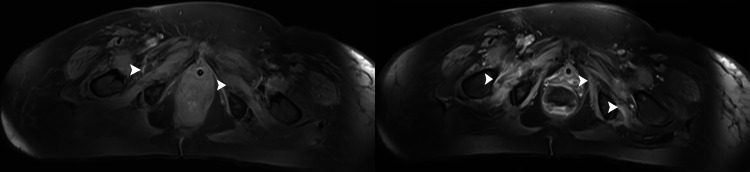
Axial fat-suppressed T1-weighted images of the pelvis. The images show (a) muscle atrophy and (b) gadolinium-enhancement (arrowheads).
